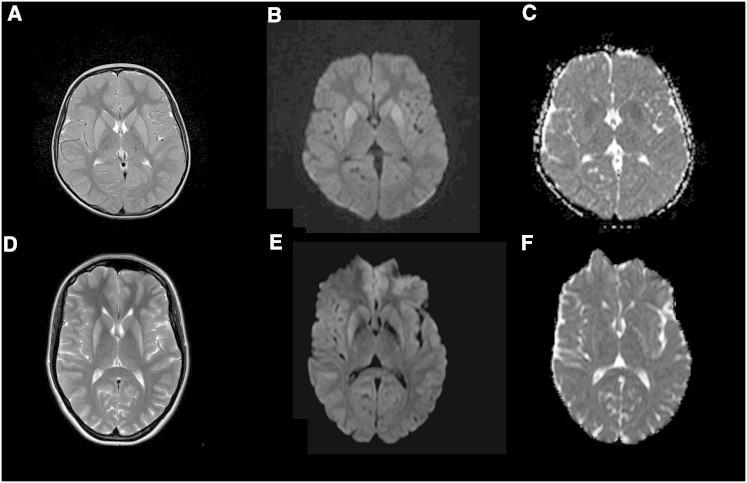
MRI Features Associated with Dominant *PDE10A* Mutations Axial MRI of individuals 1 (A–C) and 2 (D–F). T2-weighted imaging (A and D) and diffusion-weighted imaging (DWI; B and E) showed increased signal intensity within the striatum. In individual 1, the putamen and caudate nucleus appeared slightly swollen (A), and high signal on DWI (B) was confirmed to represent abnormal restricted diffusion on the ADC map (C). In individual 2, the abnormal signal was principally located in the postero-lateral putamina, which also appeared atrophic (D). There was no corresponding restriction of diffusion on the ADC map (F), and appearances suggested a more chronic disease stage.
